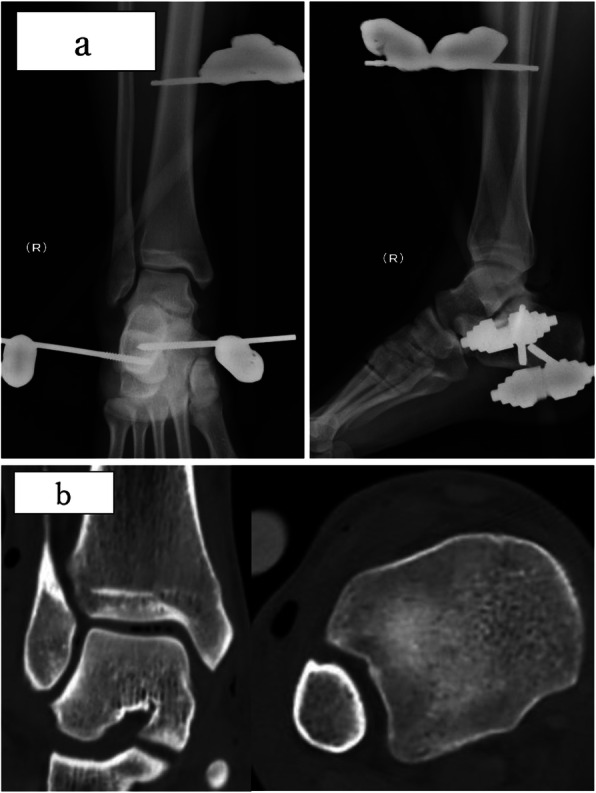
a Plain x-ray image obtained after reduction was performed using external fixation. Reduction was excellent and the ankle was stabilized in a neutral position (ankle 0° position). b CT performed after reduction revealed no malleolar fractures and normal tibia-fibula distance
